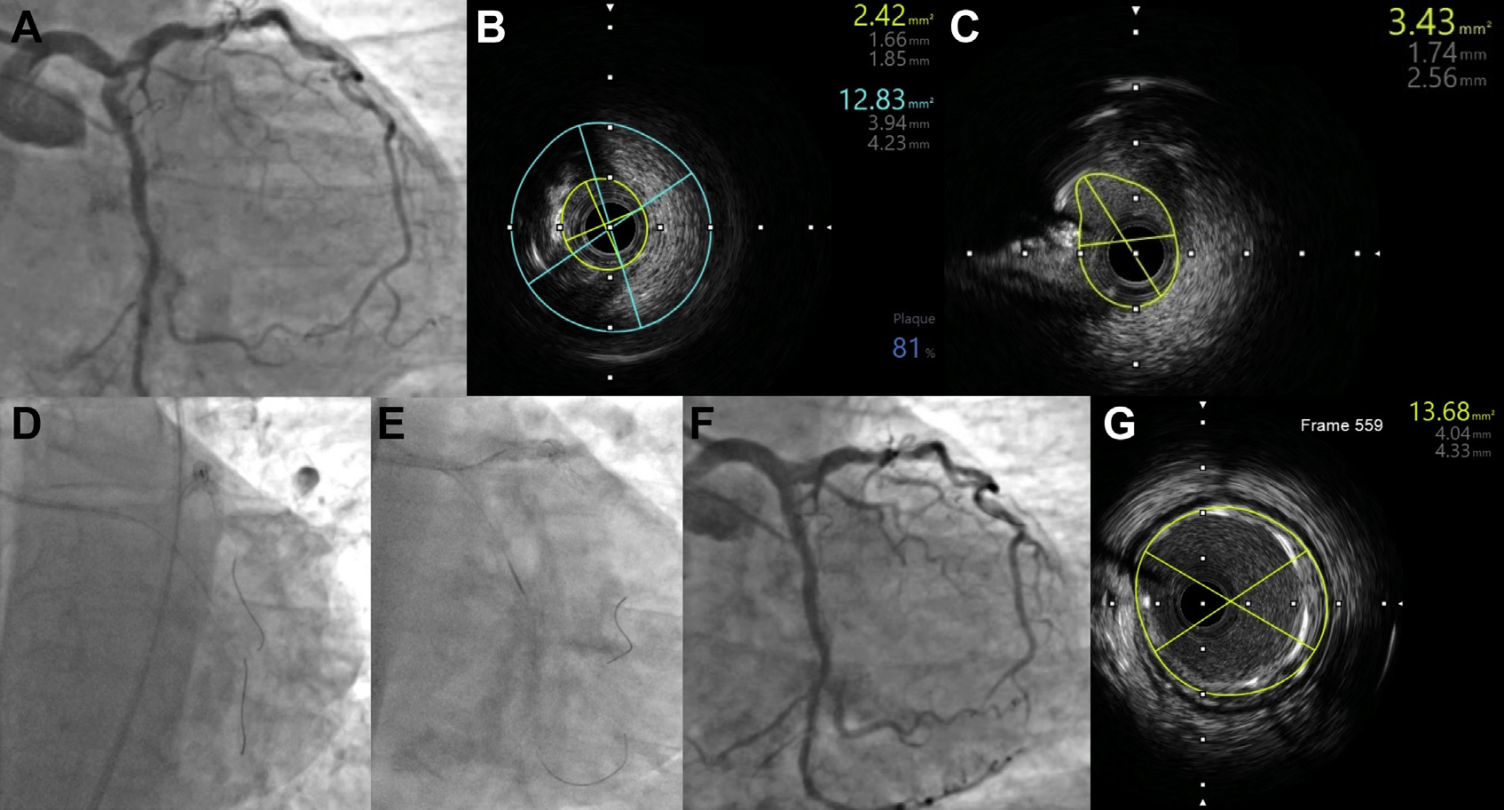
Orbital atherectomy of calcified left main bifurcation lesion. (A) Initial LM lesion, (B) initial LAD IVUS, (C) initial LCX IVUS, (D) OA of LM-LCX, (E) OA of LM-LAD, (F) final angiographic result, (G) final LM IVUS. IVUS, intravascular ultrasound; LAD, left anterior descending artery; LCX, left circumflex artery; LM, left main; OA, orbital atherectomy.
